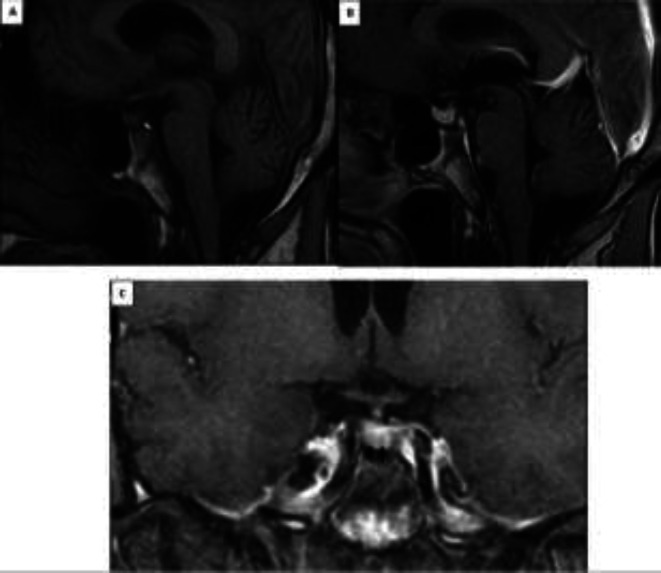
Magnetic resonance imaging of the pituitary gland. A. Sagittal view. Filiform neurohypophysis is observed with decrease intensity (white arrow) in the T1 sequence without contrast. B and C. Sagittal and coronal view. Two microadenomas of 4×2
